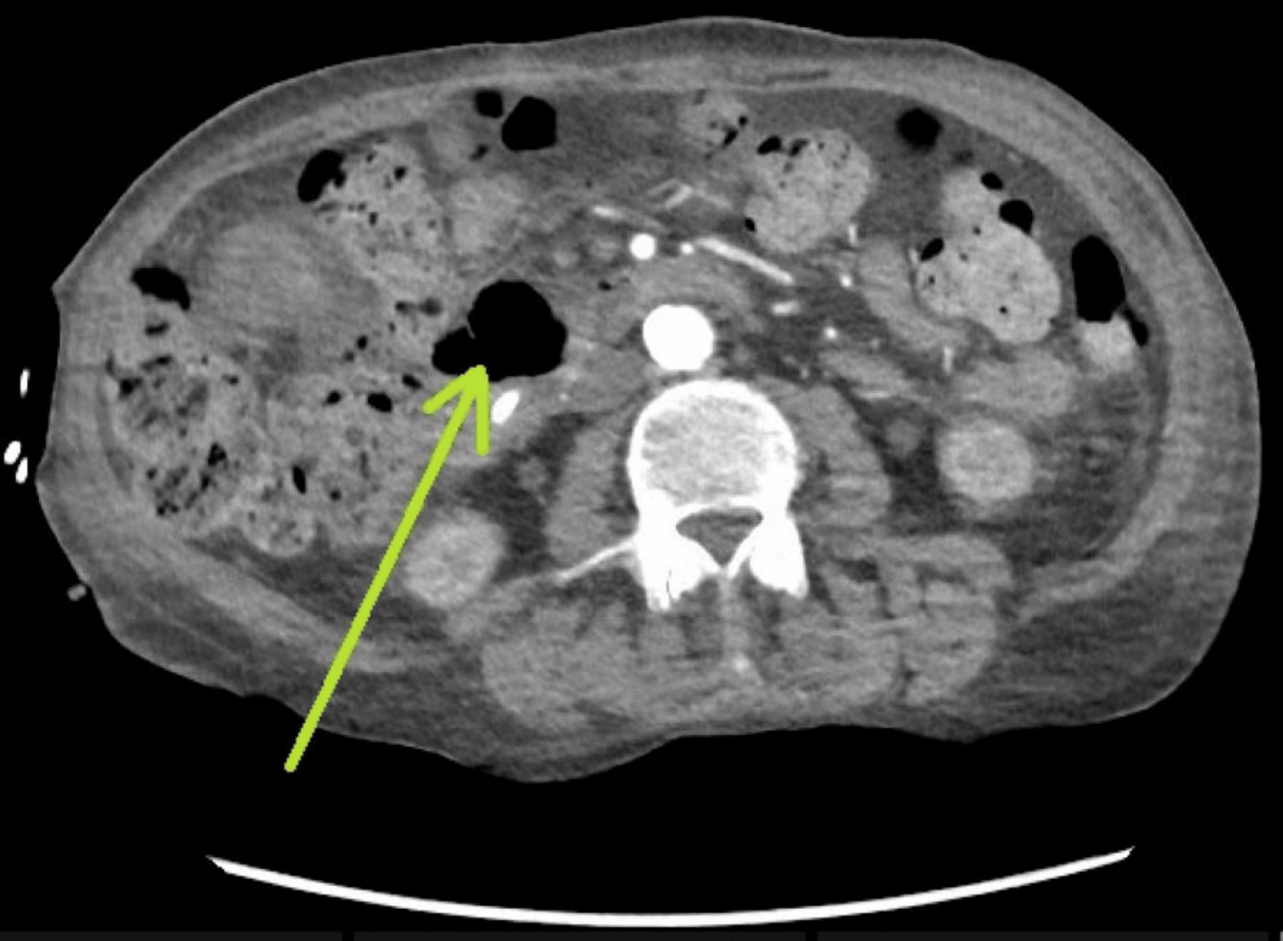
Axial contrast-enhanced CT of the abdomen showing a periampullary duodenal diverticulum compressing the distal common bile duct.
